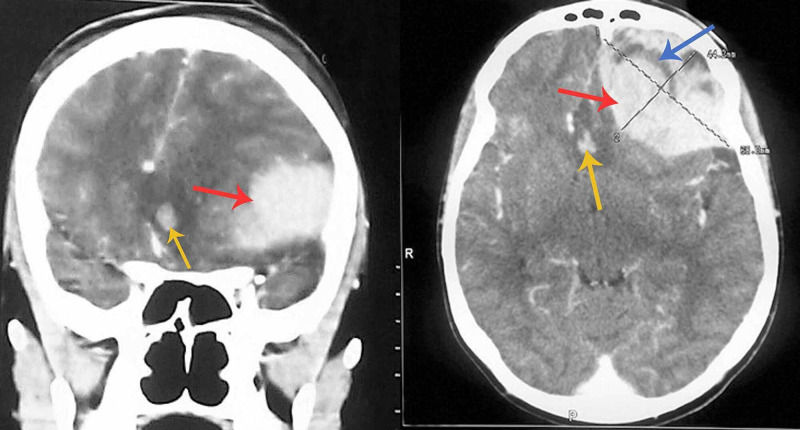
Patient's head CT scan showing left frontal epidural bleed (red arrow) and the swirl sign (blue arrow) with mass effect; and corpus callosal bleed (yellow arrow). (Left image: coronal view. Right image: axial view) CT - Computerized Tomography
